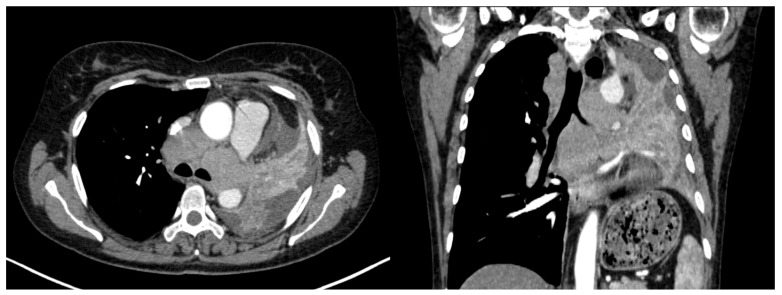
Axial and coronal CT of a left central SQCC with multiple mediastinal lymph nodes involving all mediastinal lymph stations.
